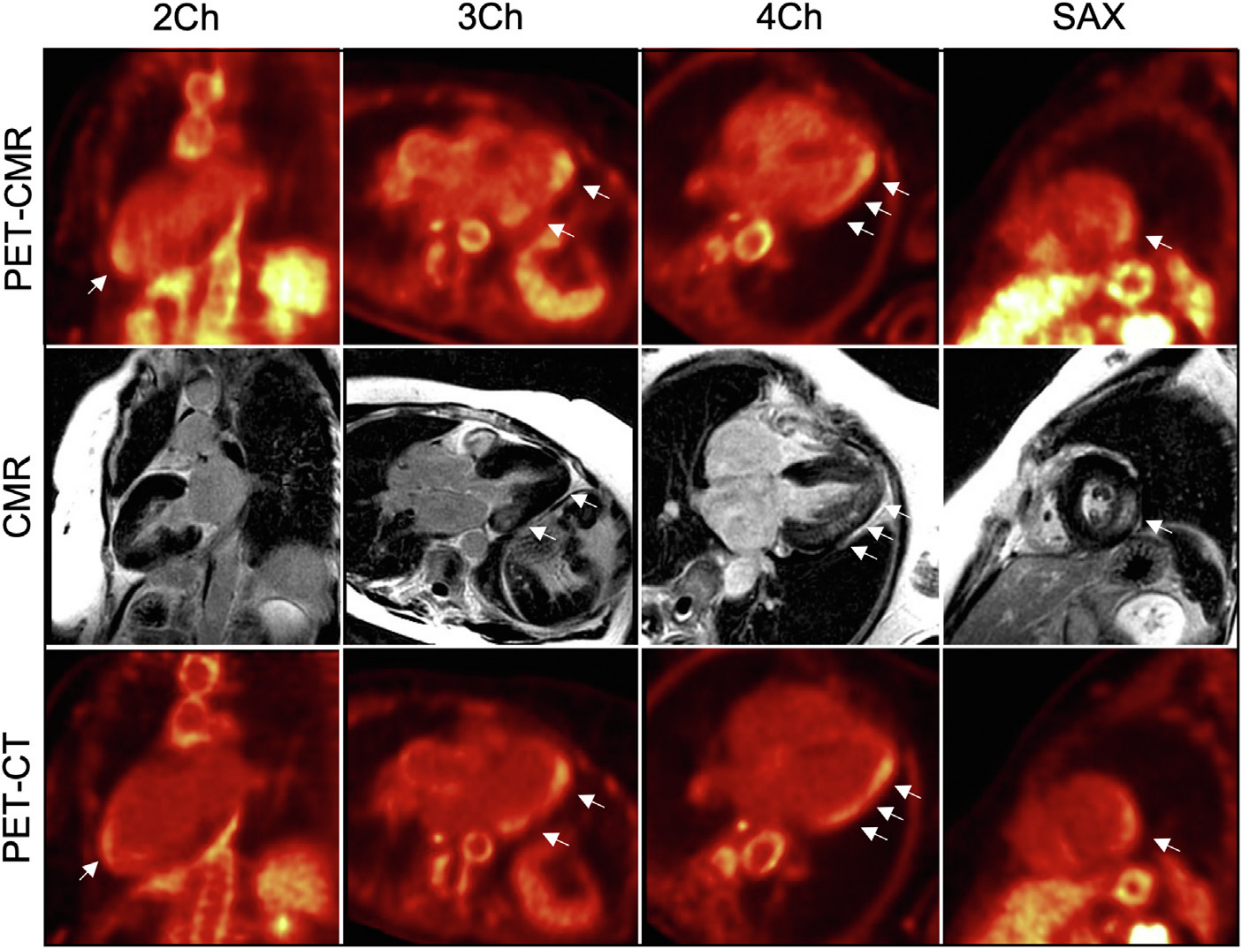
Case #1 Hybridized Images (Top row) [^18^F]-fluorodeoxyglucose ([^18^F]-FDG) positron emission tomography (PET) with cardiac magnetic resonance (CMR). (Middle row) Cardiac magnetic resonance. (Bottom row) [^18^F]-FDG positron emission tomography with computed tomography (CT). On the [^18^F]-FDG positron emission tomography with cardiac magnetic resonance and [^18^F]-FDG positron emission tomography with computed tomography images, the arrows indicate areas of increased [^18^F]-FDG uptake. On the cardiac magnetic resonance images, the arrows indicate areas of late gadolinium enhancement. Individual modality images rather than hybridized images are presented throughout to facilitate visualization of the abnormalities identified with each modality. 2Ch = 2-chamber view; 3Ch = 3-chamber view; 4Ch = 4-chamber view; SAX = short-axis view.
